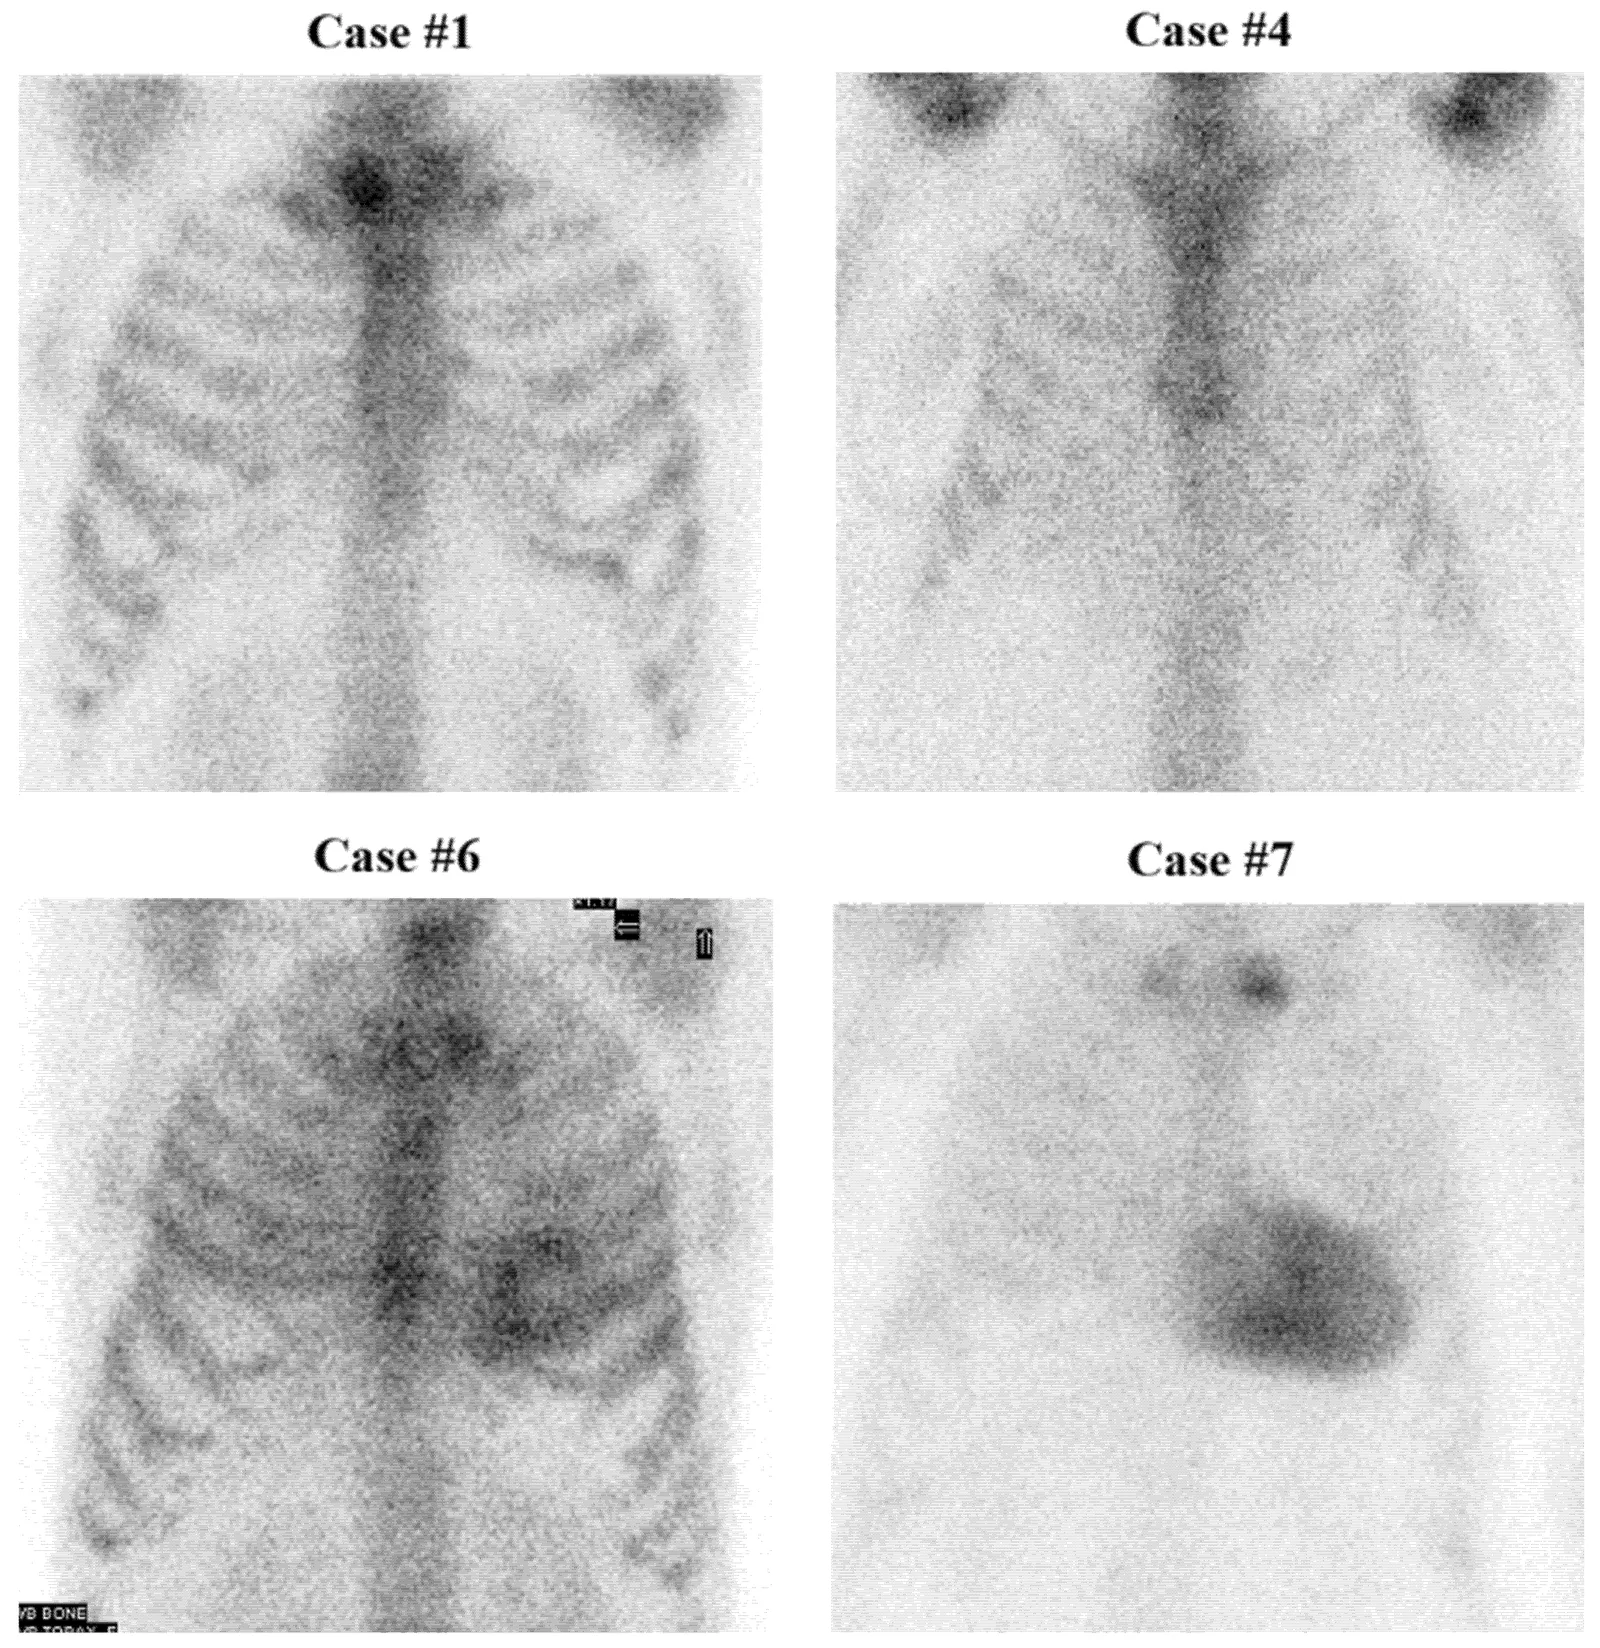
Cardiac scintigraphy with ^99m^Tc-DPD of Cases #1, #4, #6, and #7.
